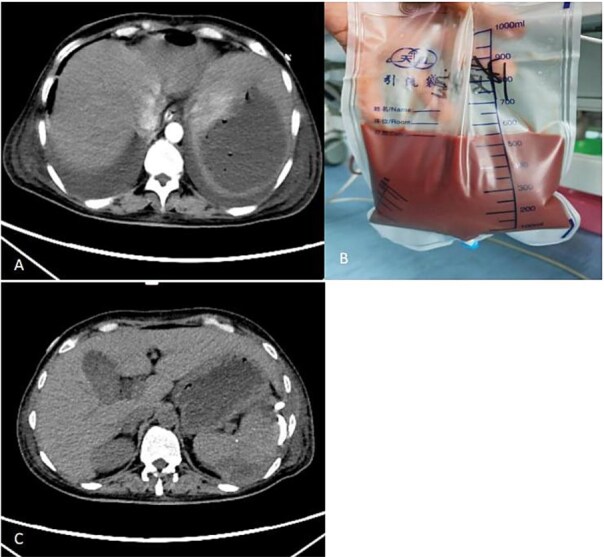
Enhanced CT scan after laparoscopic examination (A). Image of the drainage fluid obtained after spleen puncture (B). CT scan taken 6 days after the puncture and drainage of the spleen abscess (C).
